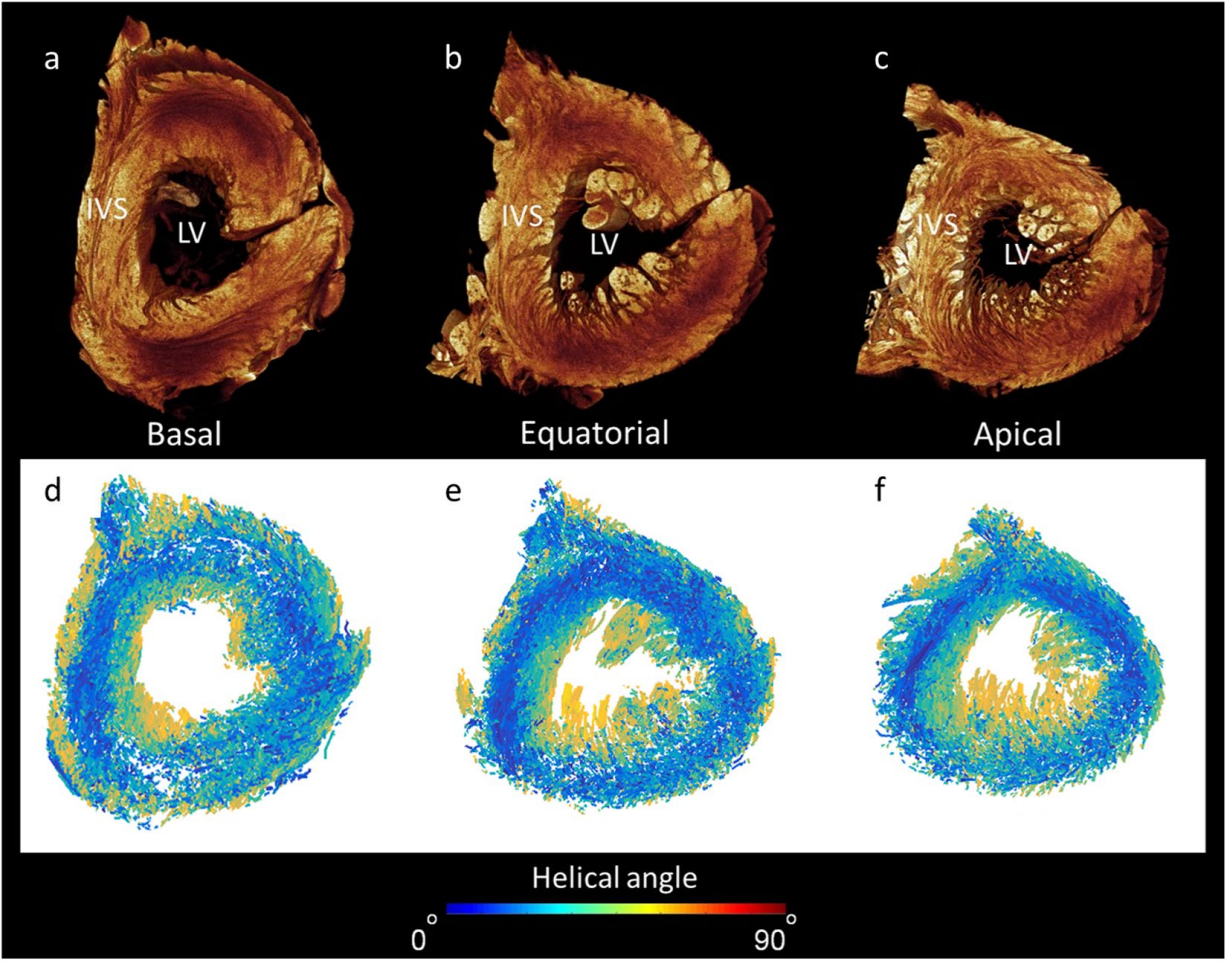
Extraction of cardiomyocyte orientation in the human left ventricle. Panels a–c show volume renderings in short-axis view taking from the basal, equatorial, and apical regions. Panels d–f show corresponding cardiomyocyte orientation in which the absolute helical angles derived from the CT dataset are coded in colour (see colour map). Note the right ventricular free wall is removed from view. IVS- interventricular septum, LV- left ventricular cavity.
